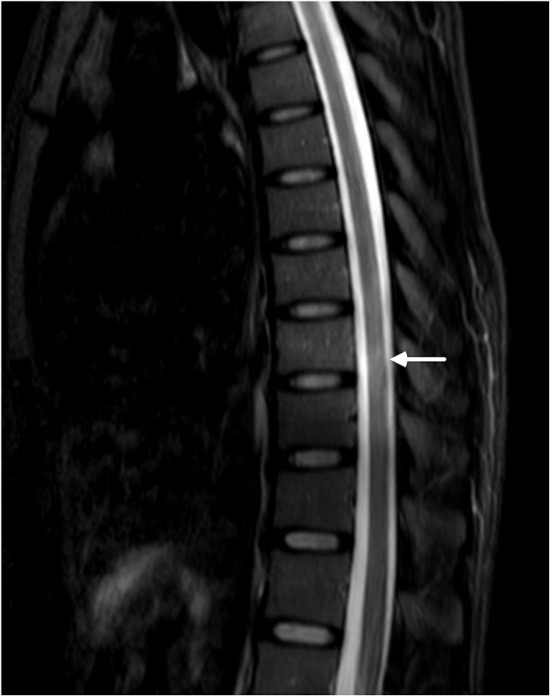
MRI of the lower thoracic spine showed T2 hyperintense lesions from T7 through T9 (arrow). However, the signal was blurry, and we considered it to be undetermined.
